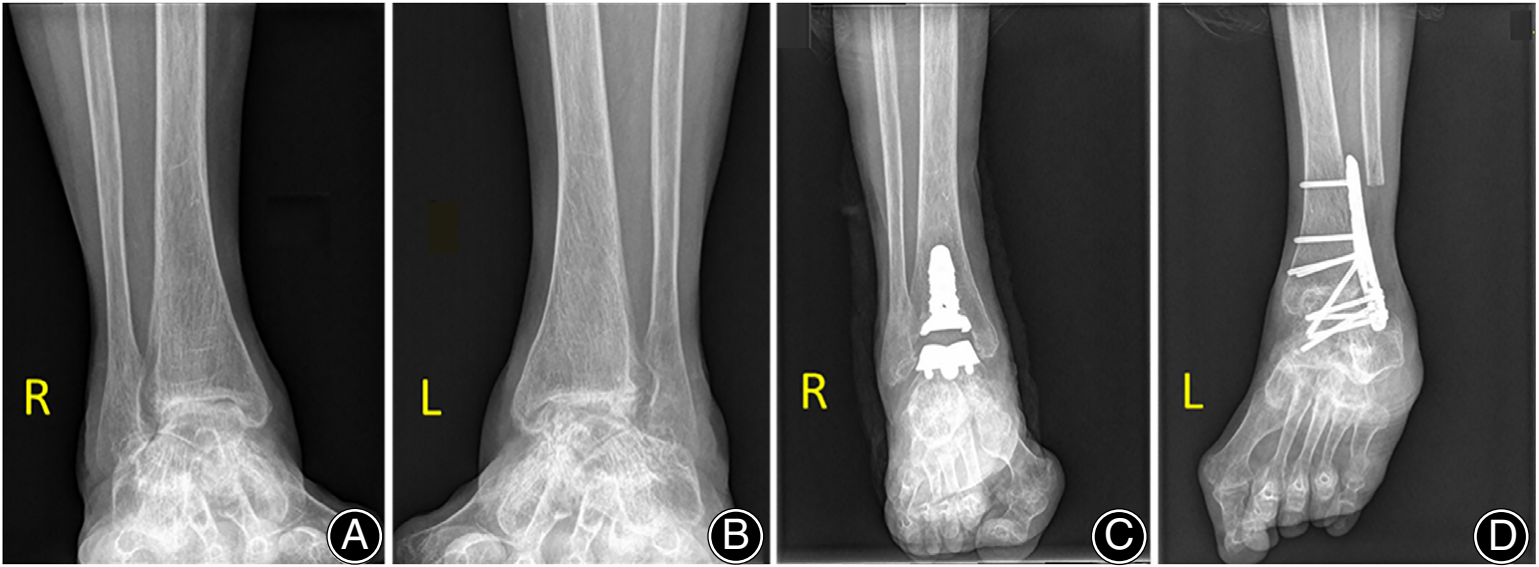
Preoperative and postoperative weightbearing radiographs of the arthroplasty and arthrodesis procedures. The patient was a 61‐year‐old female diagnosed with bilateral rheumatoid arthritis (A, B). Anterior radiographs of INBONE II and arthrodesis (C, D)
